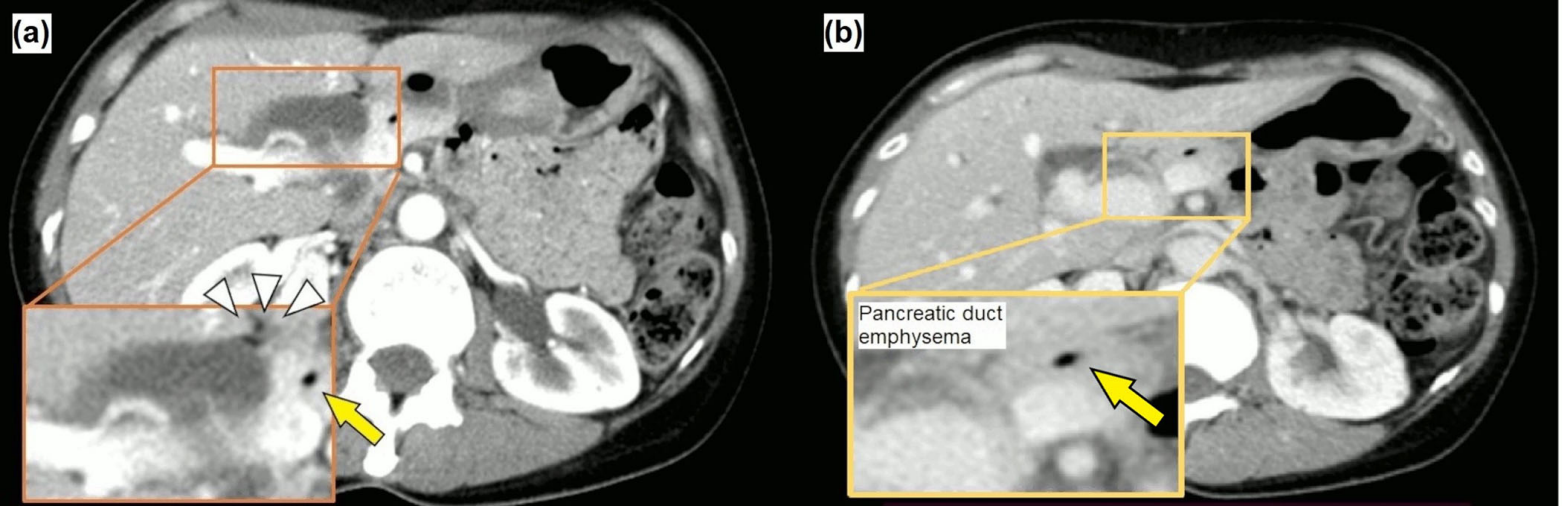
Abdominal computed tomography showed pancreatic duct emphysema (yellow arrow in (a) and (b)) and pneumobilia (white arrowheads in (a)) despite the presence of an untreated duodenal papilla
